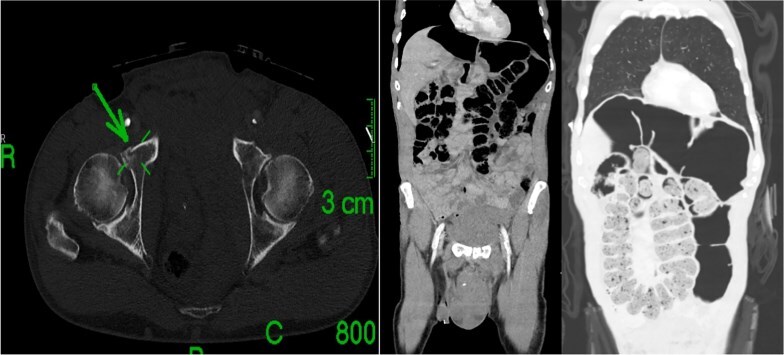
Trauma computed tomography (CT) of thorax and abdomen after initial trauma.
